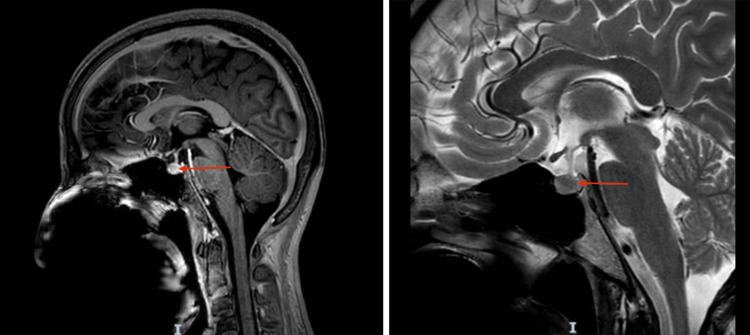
Sagittal section of MRI brain showing a normal pituitary gland
